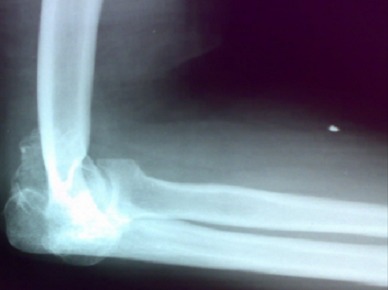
Radiographie de profil montrant la radio-ulnaire en place
